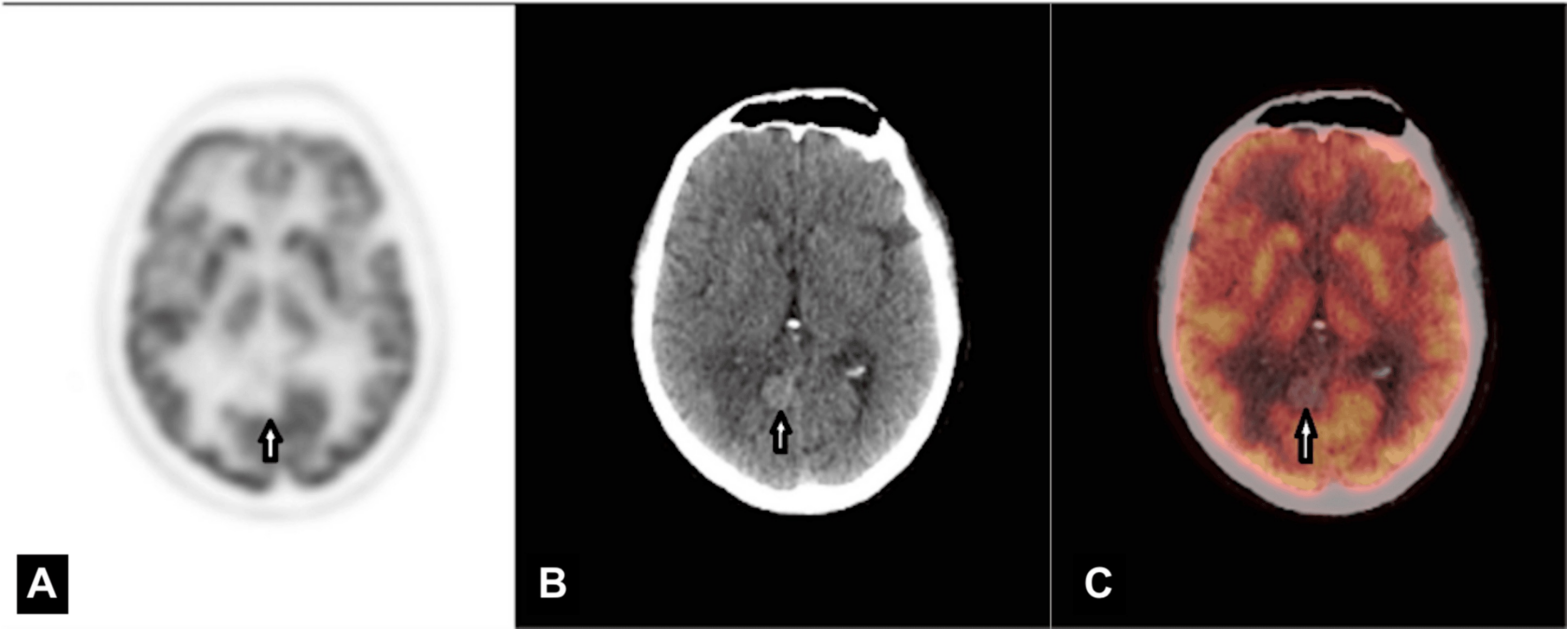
Axial FDG PET (A), CT (B), and fusion PET/CT (C) sections of the right occipital lesion demonstrating no significant FDG metabolic activity (white arrows), consistent with an iodine-FDG mismatch. FDG PET: fluorodeoxyglucose positron emission tomography; CT: computed tomography
